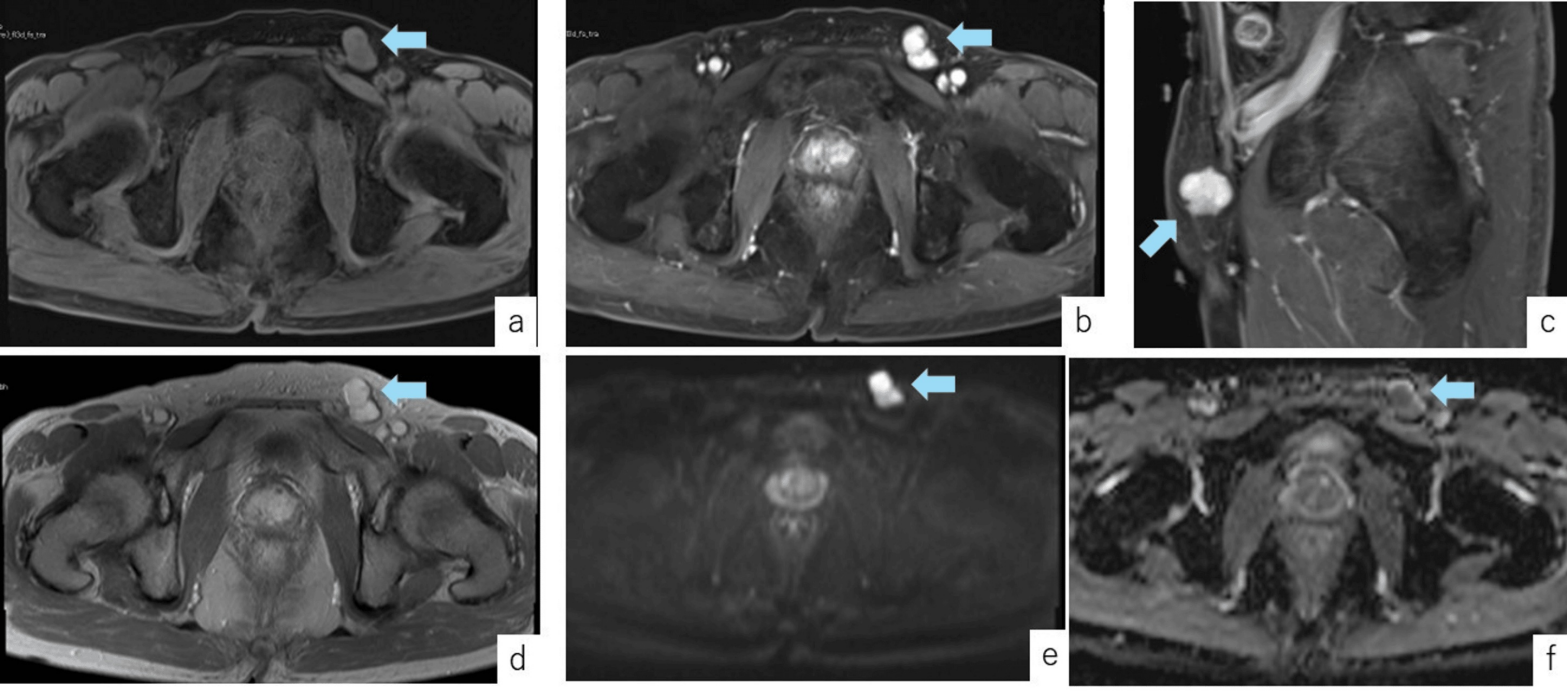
Preoperative Findings on Contrast-Enhanced MRI Contrast-enhanced MRI revealed a subcutaneous tumor in the left inguinal region, approximately 20×13 mm in size. The dynamic contrast-enhanced fat-suppressed T1-weighted sequence at 90 seconds showed heterogeneous enhancement suggestive of a lymph node tumor. Additionally, slight enlargement of the prostate and posterior bladder wall suggested the possibility of metastatic lymph node involvement. a. Fat-suppressed T1-weighted MRI (Pre-contrast, Axial View): The tumor (arrow) was located within the soft tissue. b. Fat-suppressed T1-weighted MRI (Post-contrast, Dynamic, 90 seconds, Axial View): The tumor (arrow) showed lobulated enhancement. c. Fat-suppressed T1-weighted MRI (Post-contrast, Dynamic, 90 seconds, Sagittal View): The tumor (arrow) was located below the superficial fascia. d. T2-weighted MRI Image: The tumor (arrow) was suspected to be a tumor as there was enlargement of the prostate and bladder. e. Diffusion-Weighted MRI : The tumor (arrow) showed high signal intensity. f. MRI ADC Map: The tumor (arrow) showed low signal intensity. MRI: magnetic resonance imaging, ADC: apparent diffusion coefficient
